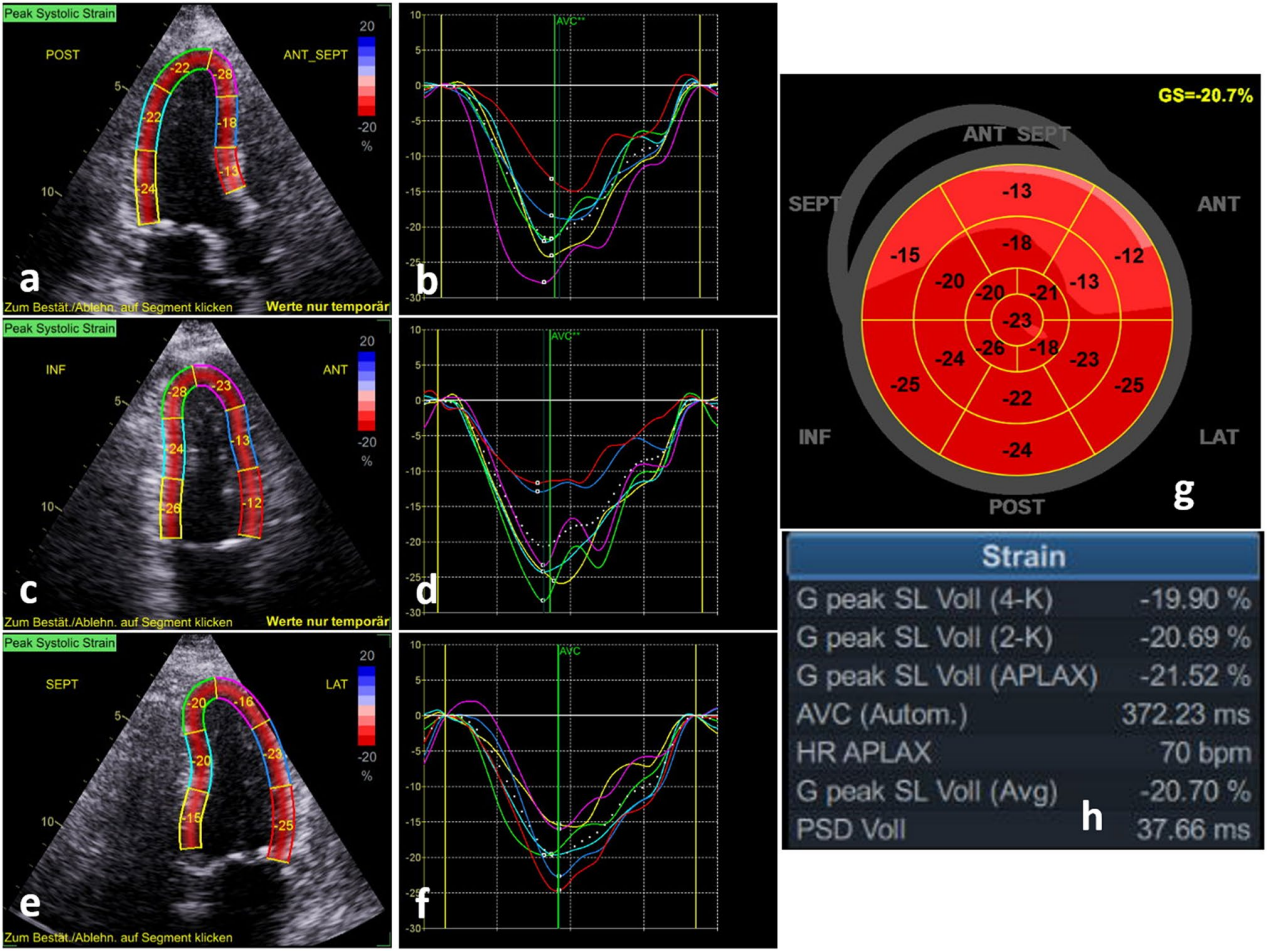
Follow-up echocardiographic deformation imaging after 2 months. Peak systolic strain and corresponding regional longitudinal strain curves tracked in the apical long axis view (a, b), the 2-chamber view (c, d) and the 4-chamber view (e, f). The bull’s eye plot of the typical pattern of regional longitudinal strain of the left ventricle is presented in g, the respective strain data are shown in (h)
